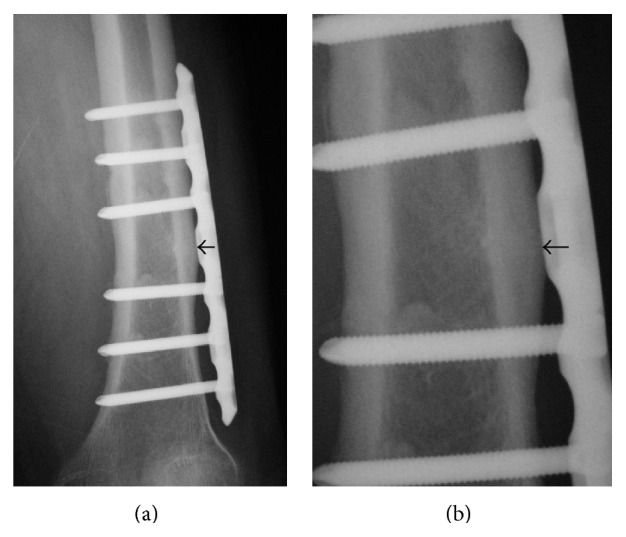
Anteroposterior radiographs of the left femur 3 months after surgical treatment. (b) is a magnified view of (a). The horizontal line over the outer cortical bone (black arrows) had almost disappeared.
